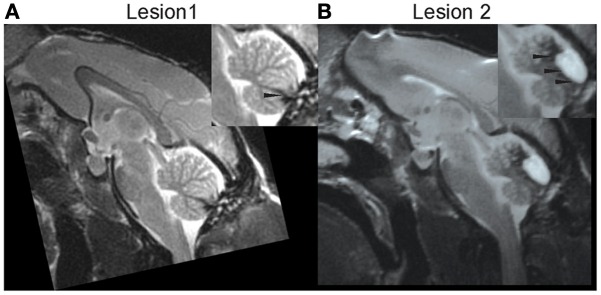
High resolution midline sagittal MRI slice after lesion 1 (A) and after lesion 2 (B). Adopted from Ignashchenkova etal. (2009). Further technical details are provided there.
